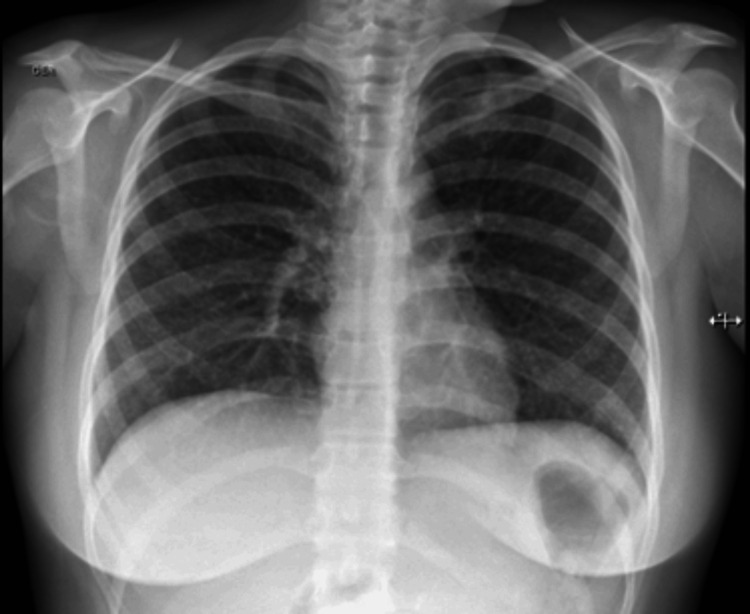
Chest X-ray showing diffuse micronodular radiopacities on both lungs.
